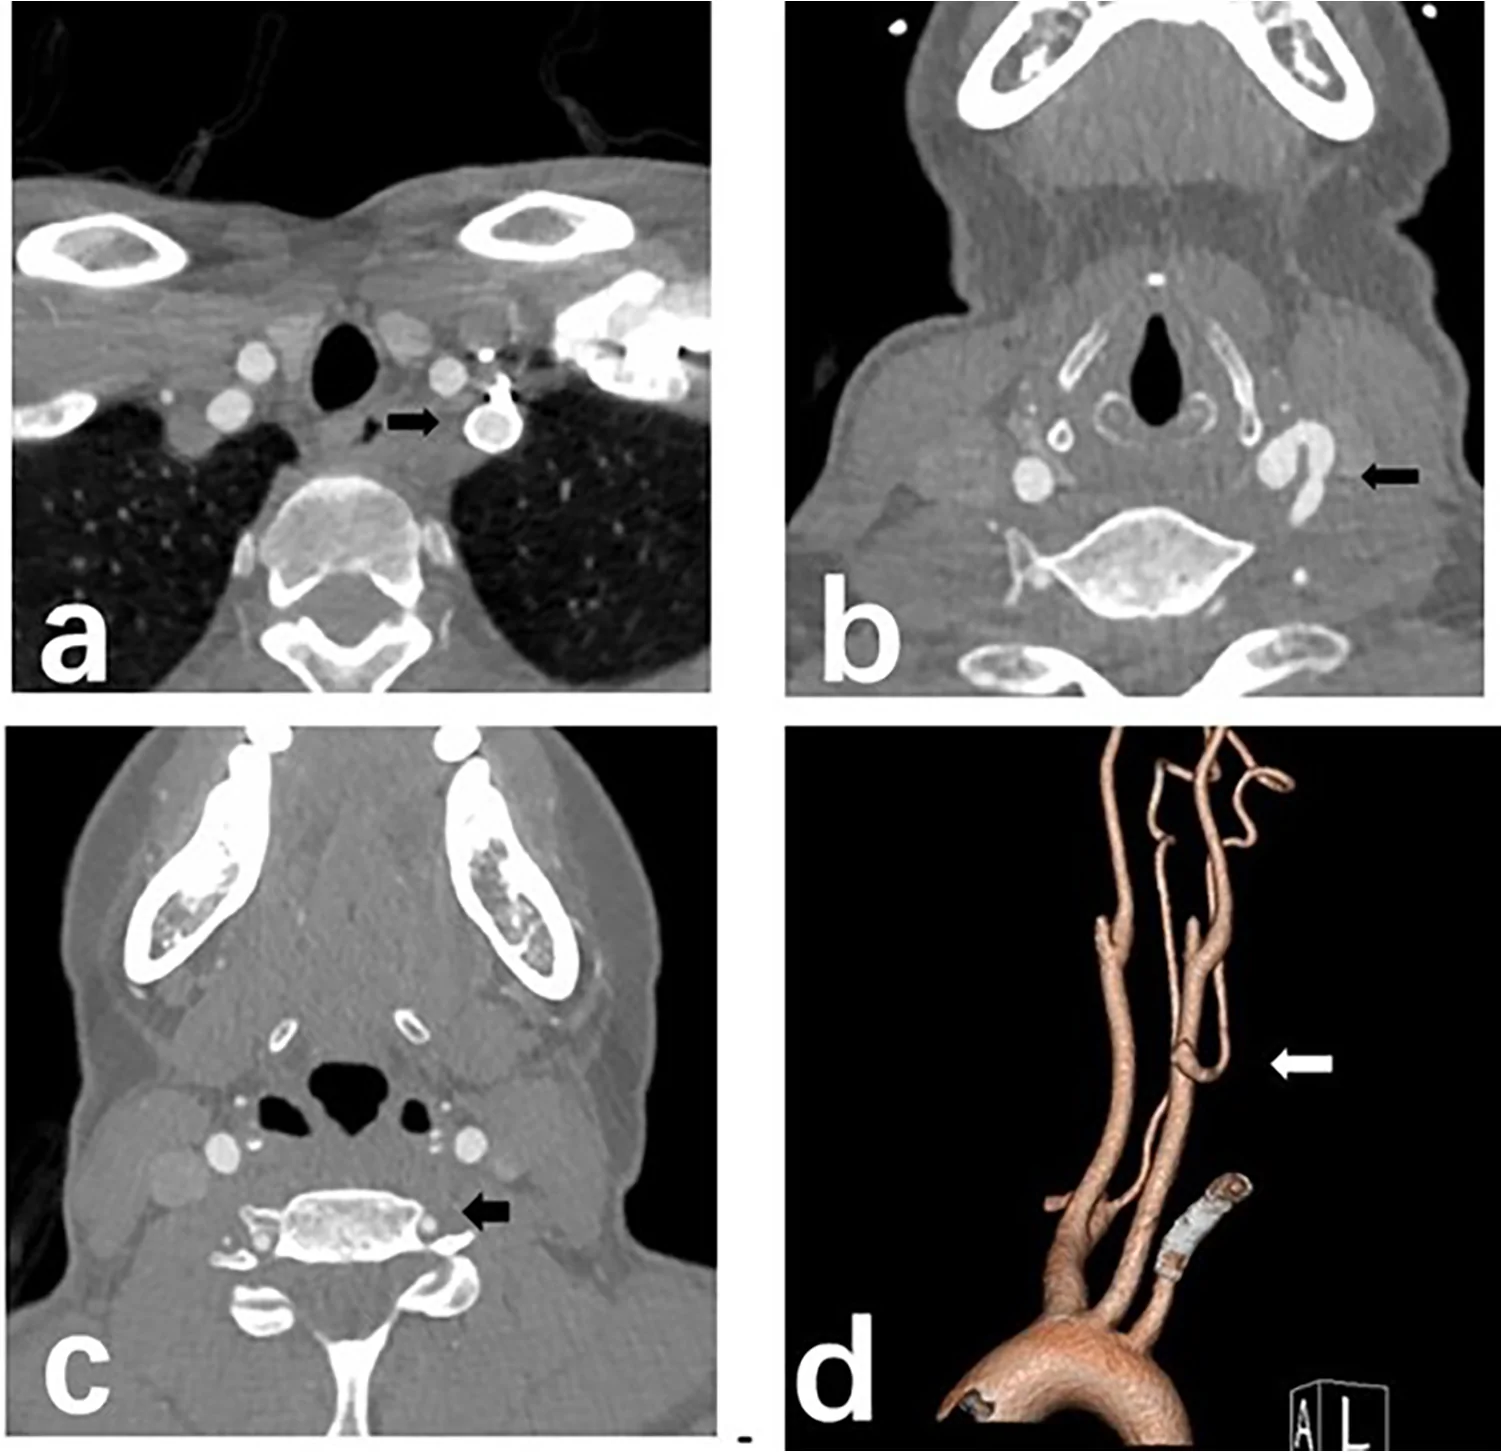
Contrast-enhanced CT and 3D-CTA images obtained 11 months after treatment. (a) Left subclavian angiogram showing subclavian artery stent patency. (b) Distal-vertebral-to-internal-carotid bypass in the cross section. (c) The anterior edge of the foramen was partly resected. (d) 3D-CTA showing the subclavian artery stent and vertebral-to-internal-carotid bypass.
